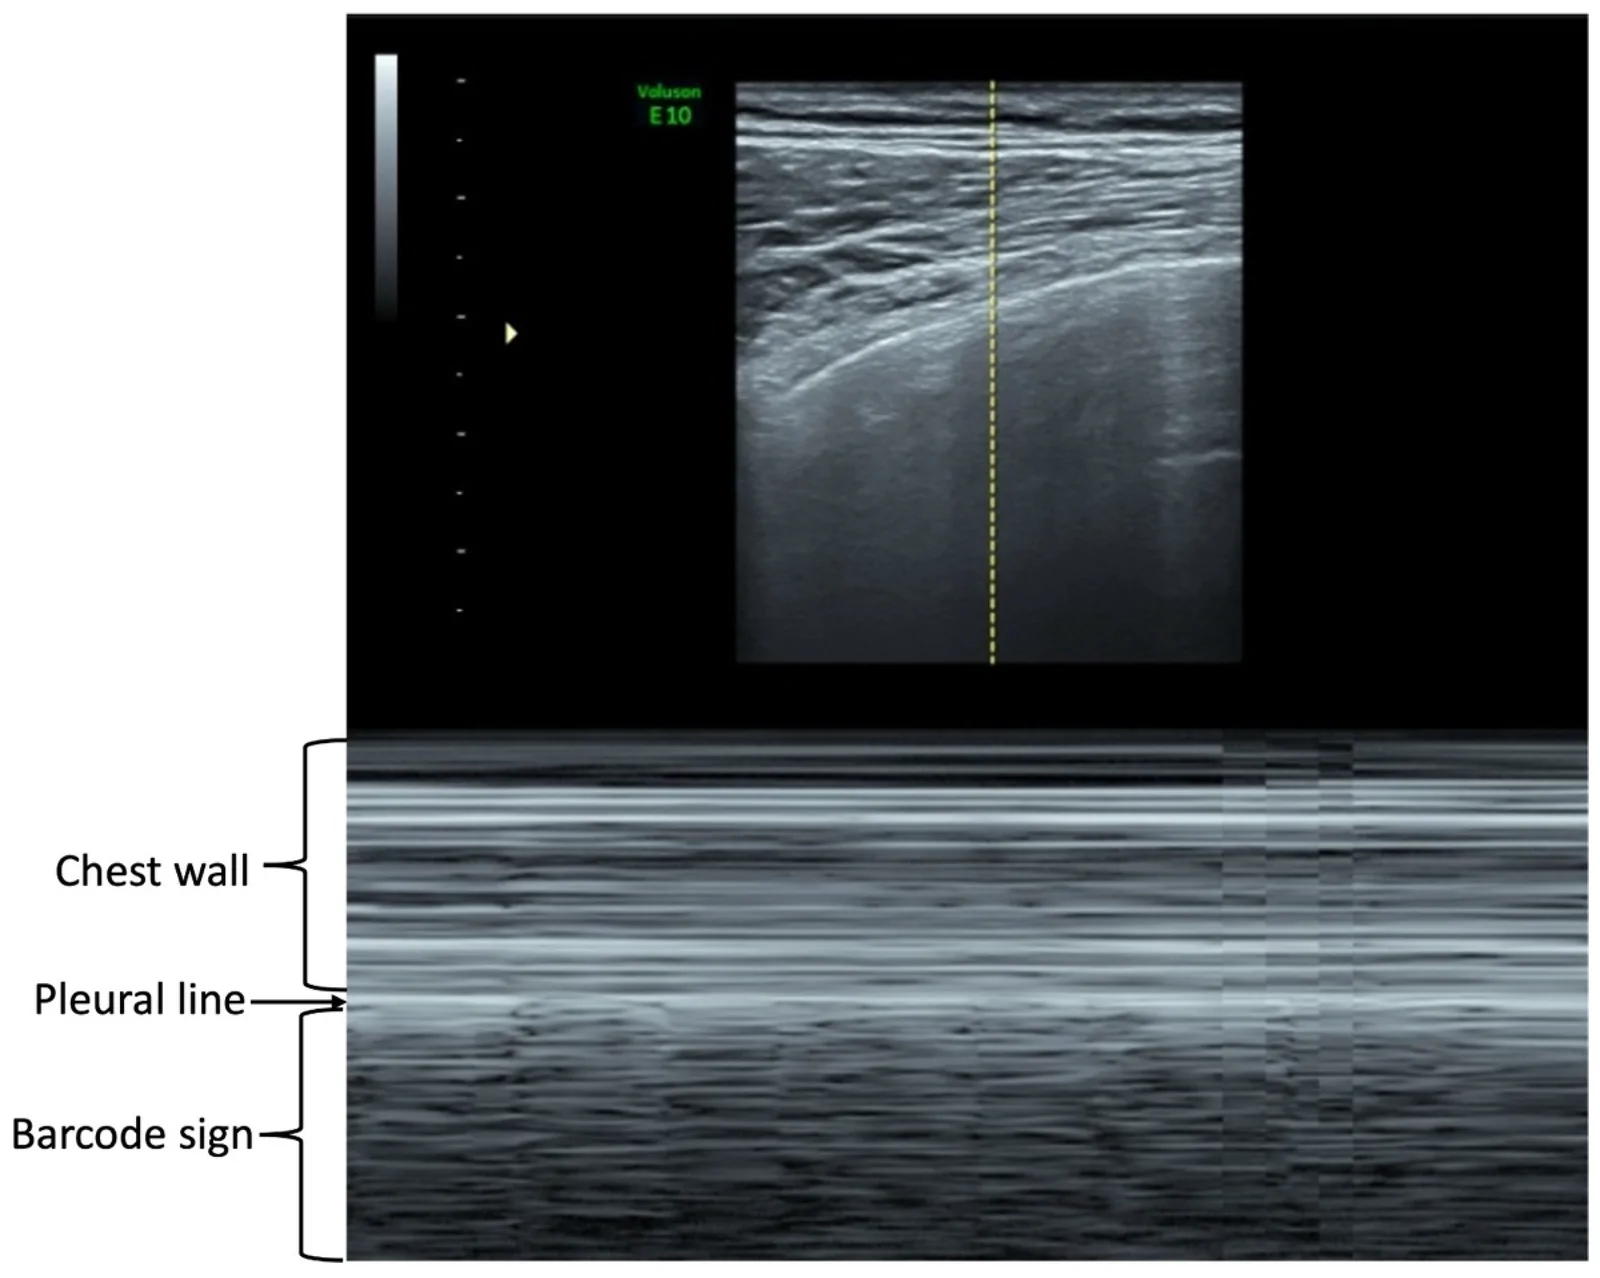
Right midaxillary M-mode transthoracic ultrasonography scan showing the absence of pleural-line motion (“barcode sign”) in a patient with severe asthma without pneumothorax. The yellow arrowhead indicates the focal zone, and the yellow dashed line indicates the sampling line used for M-mode examination.
